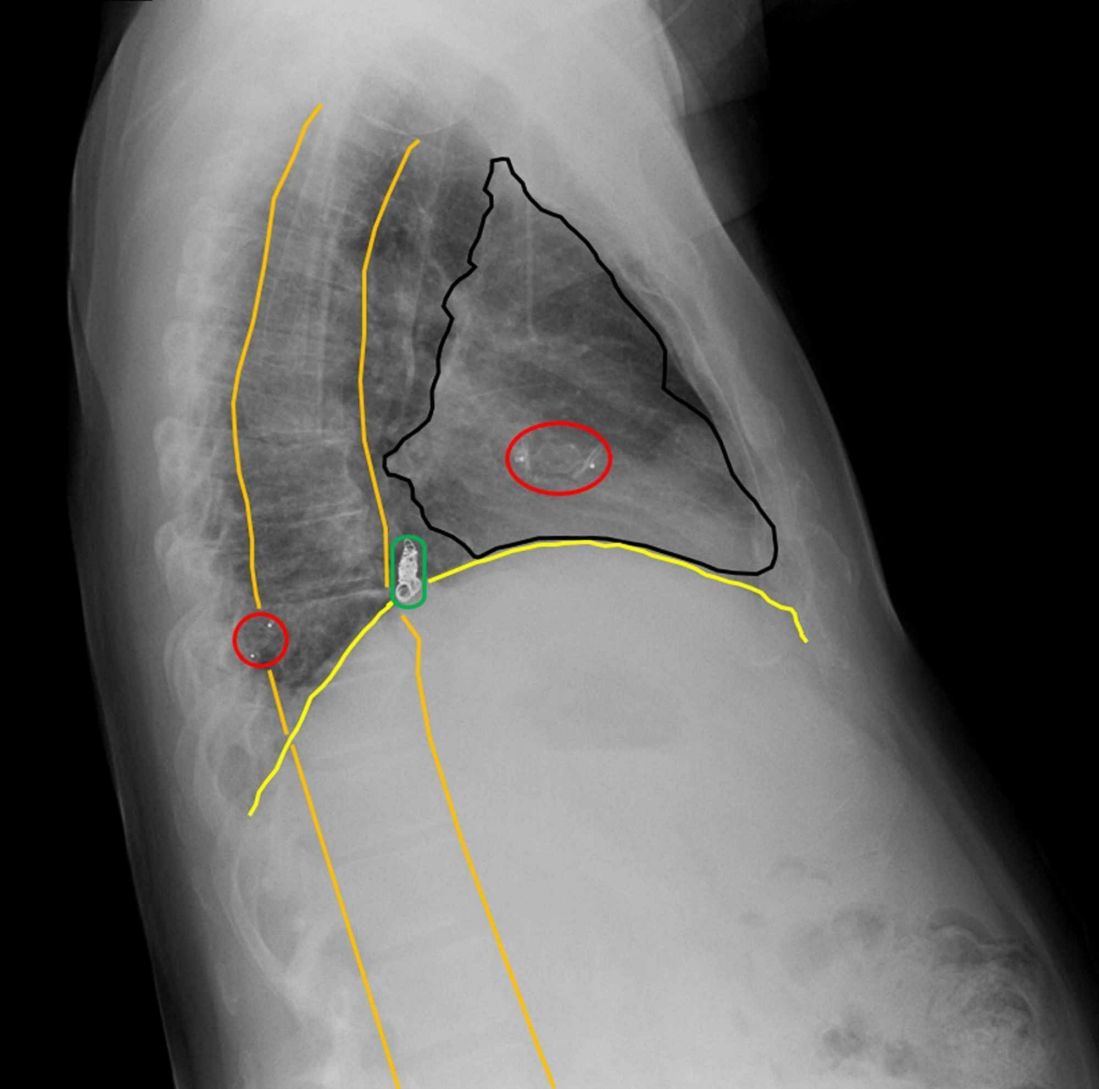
Postoperative Radiograph Showing Appropriately Positioned Embolization Devices Throughout the Chest A postoperative lateral chest radiograph demonstrates Amplatzer plugs (red circles) and Terumo detachable microcoils (green circle) at the locations of the embolized AVMs. The heart border (black outline), diaphragm (yellow line), and vertebral column (orange lines) are demonstrated in the lateral view.
